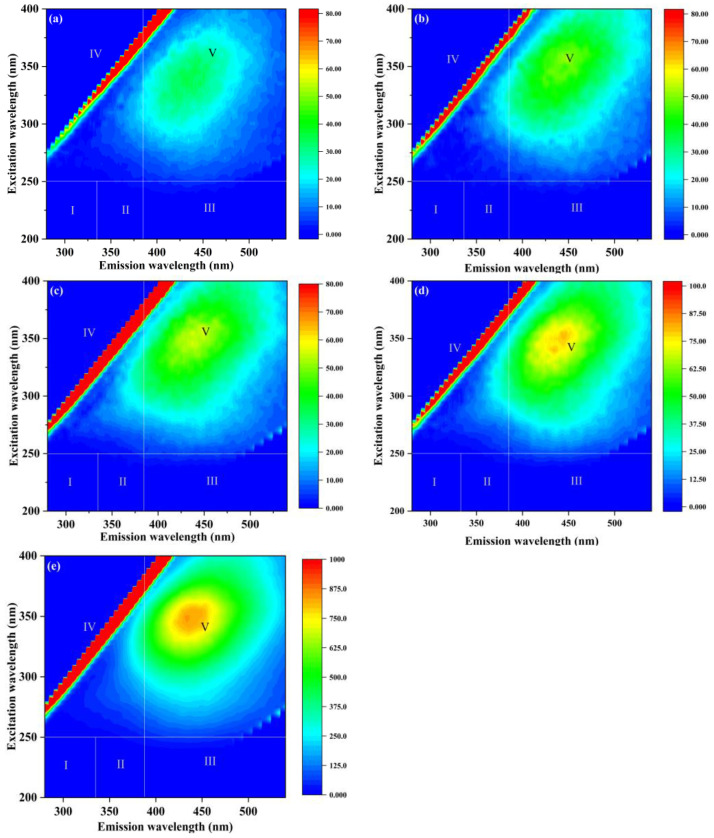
EEM spectra: (a) raw water, (b) ultrasonic eluate, (c) sodium citrate eluate, (d) NaClO eluate, and (e) NaClO + NaOH eluate.
